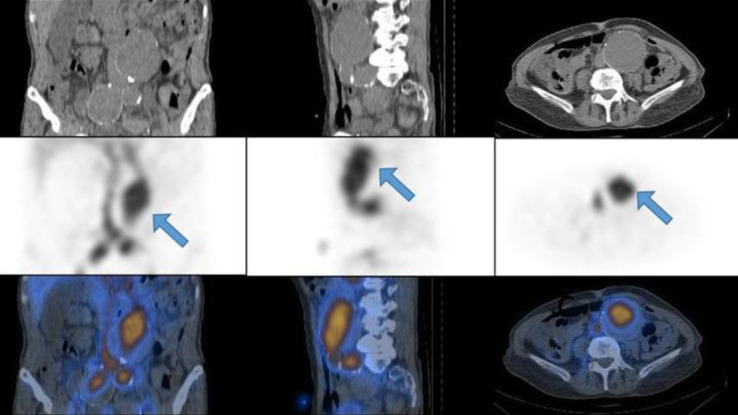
Calcified abdominal aortic aneurysm (CAAA) on SPECT/CT (arrows)
